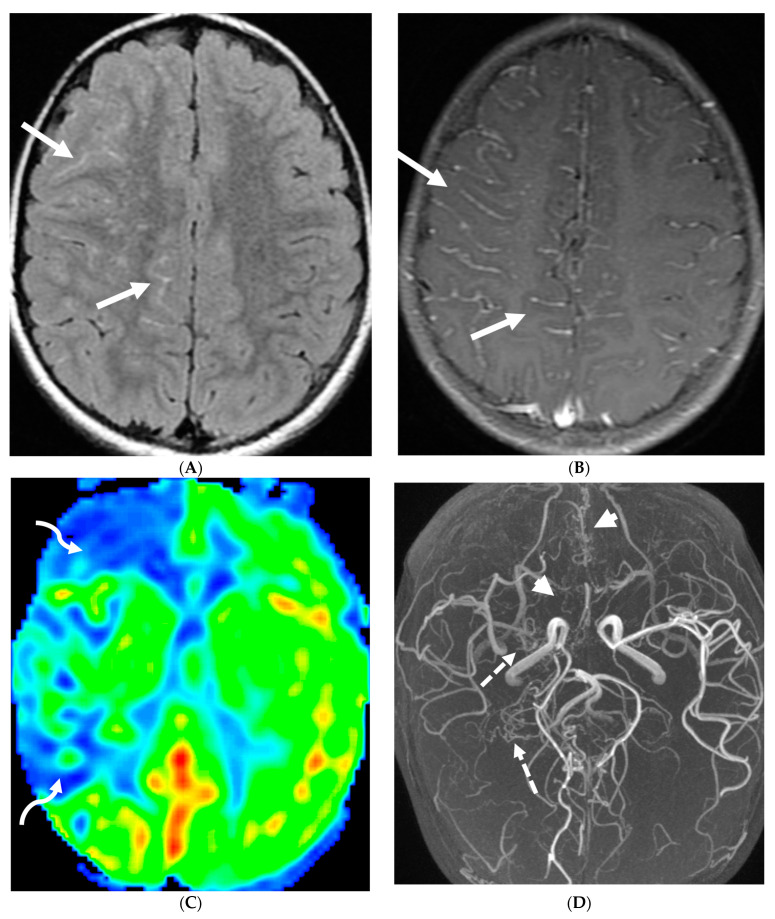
Axial FLAIR (A), MRA (B), axial ASL perfusion (C) and axial T1 post contrast (D): 7-year-old girl with Down’s syndrome: Abnormal FLAIR hyperintense signal with LME along the right cerebral convexity sulci, predominantly in the frontoparietal region representing “ivy sign” (arrows). Asymmetric decreased perfusion in the right frontal and temporal regions (curved arrows). The M1 segment of right MCA is not visualized with extensive moyamoya vessels (dashed arrows). The M2 and M3 branches of right MCA are asymmetrically attenuated. Bilateral A1 segments are not identified with extensive collateralization and diminutive caliber of A2 and A3 segments (arrow heads).
